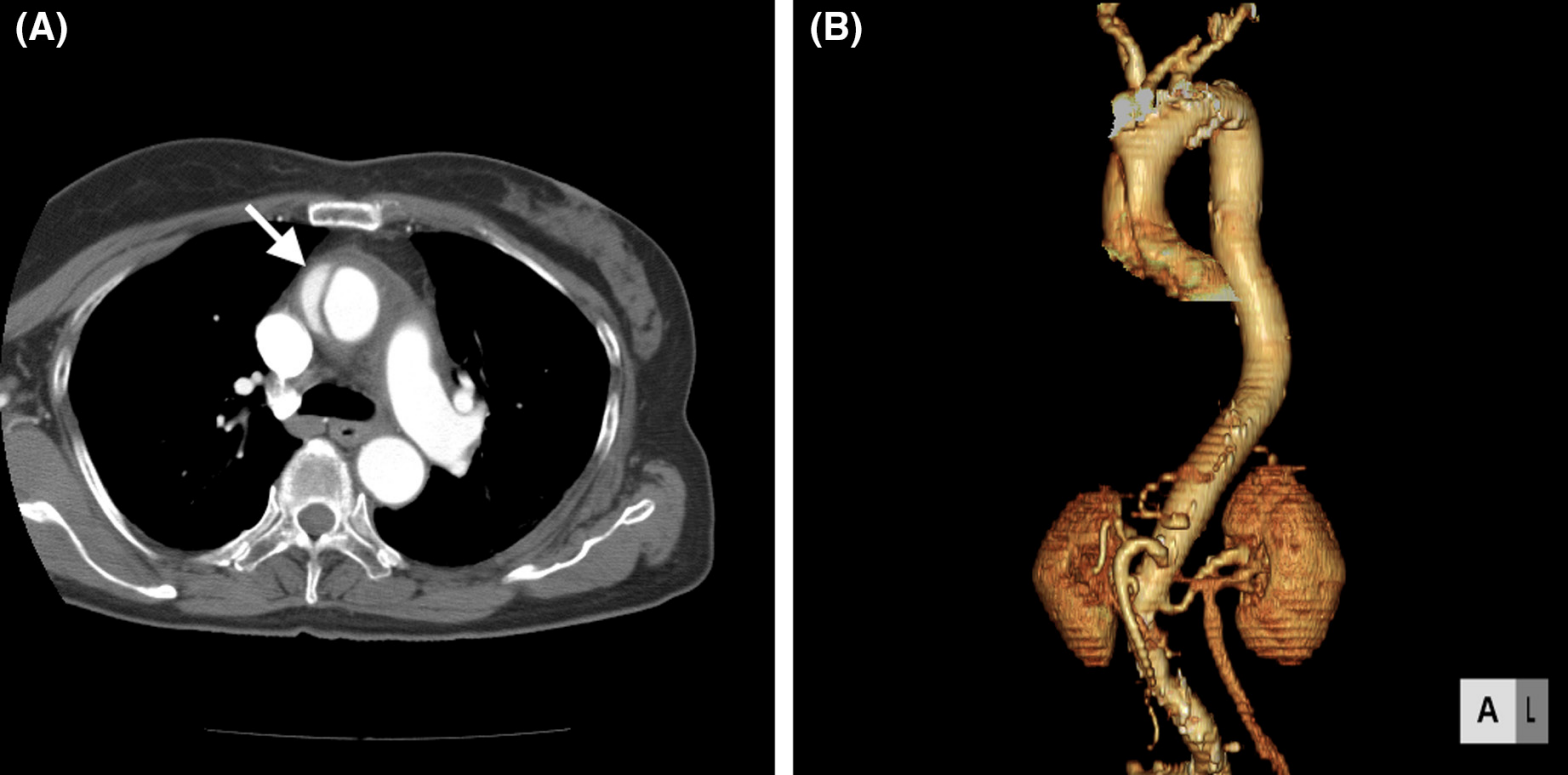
(A) Computed tomography imaging showing ascending aortic dissection with intimal flap (arrow). (B) 3D computed tomography imaging showing ascending aortic dissection.
